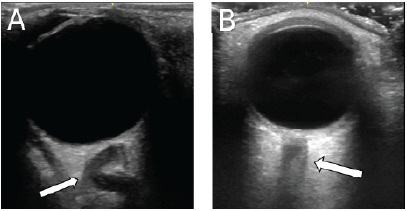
A) Point-of-care ultrasound image demonstrating tortuous optic nerve of the left eye from the patient (arrow), and B) a normal optic nerve of the left eye unrelated to the patient (arrow).
